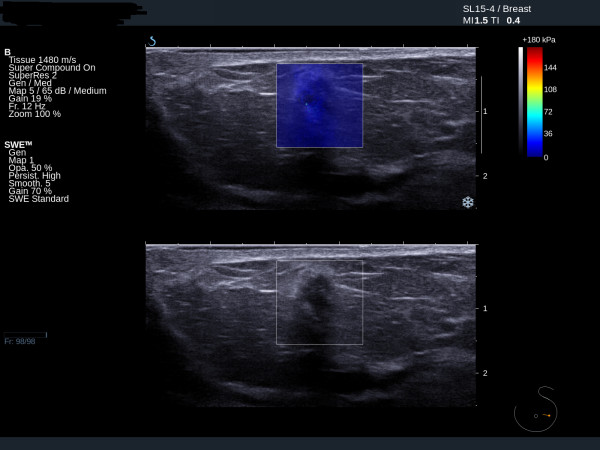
Ultrasound and elastography images of a benign fibroadenoma. Ultrasound and elastography images showing a benign fibroadenoma with suspicious greyscale ultrasound features but benign elastography features. Mean 32 kPa.
